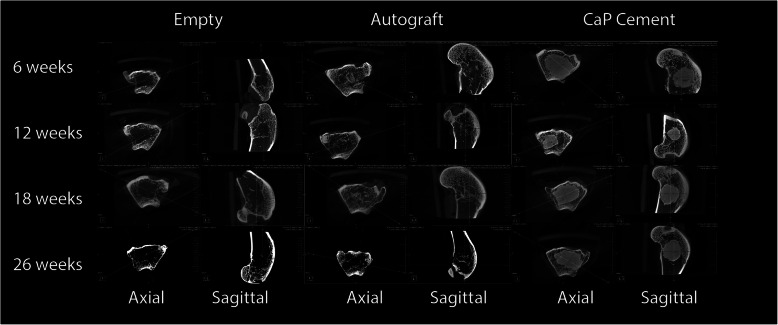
Microcomputed tomography overviews in the axial and sagittal planes for empty, autograft, and CaP material filled defects versus time. The empty defects remained empty reflecting the critical nature of the model. The autograft remodelled in the defect while the CaP material remained present with direct bone ongrowth as well as interdigitation with the host bone
